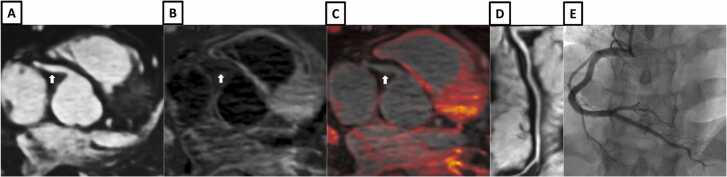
A patient with a normal RCA (white arrows). A-Bright-blood CMRA from iT2Prep-BOOST. B-Co-registered, black-blood iT2Prep-BOOST image. C-Co-registered fusion image of iT2Prep-BOOST bright-blood and black-blood datasets. D-CMRA curved multiplanar reformat of the RCA. E-invasive X-ray coronary angiography. *CMRA c*oronary magnetic resonance angiography, *RCA* right coronary artery
